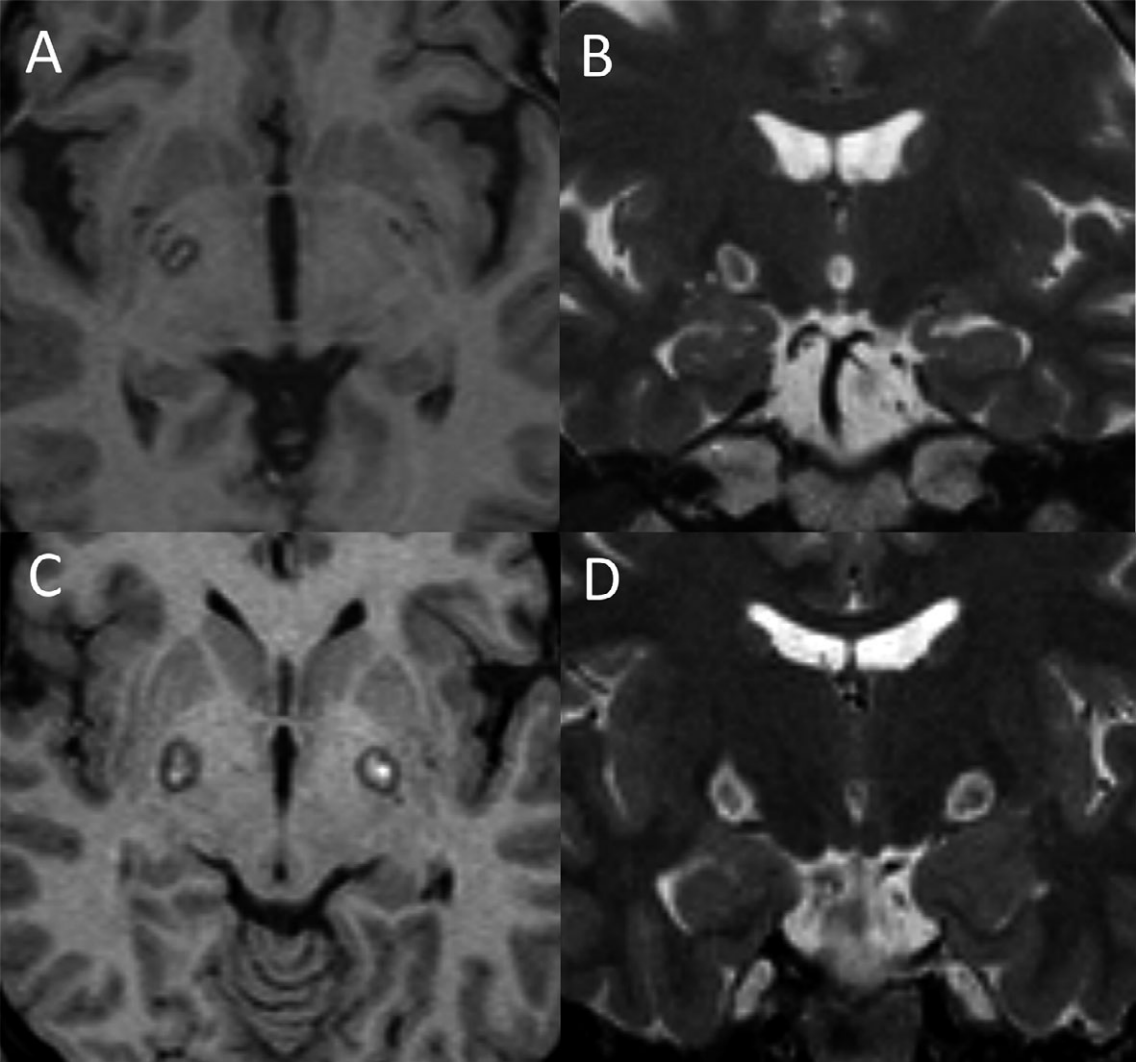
MRI images of the patients after undergoing pallidotomy. (A–B) show postoperative T1‐ and T2‐weighted MRI after undergoing unilateral pallidotomy. (C–D) show postoperative T1‐ and T2‐weighted MRI after undergoing simultaneous bilateral pallidotomy.
